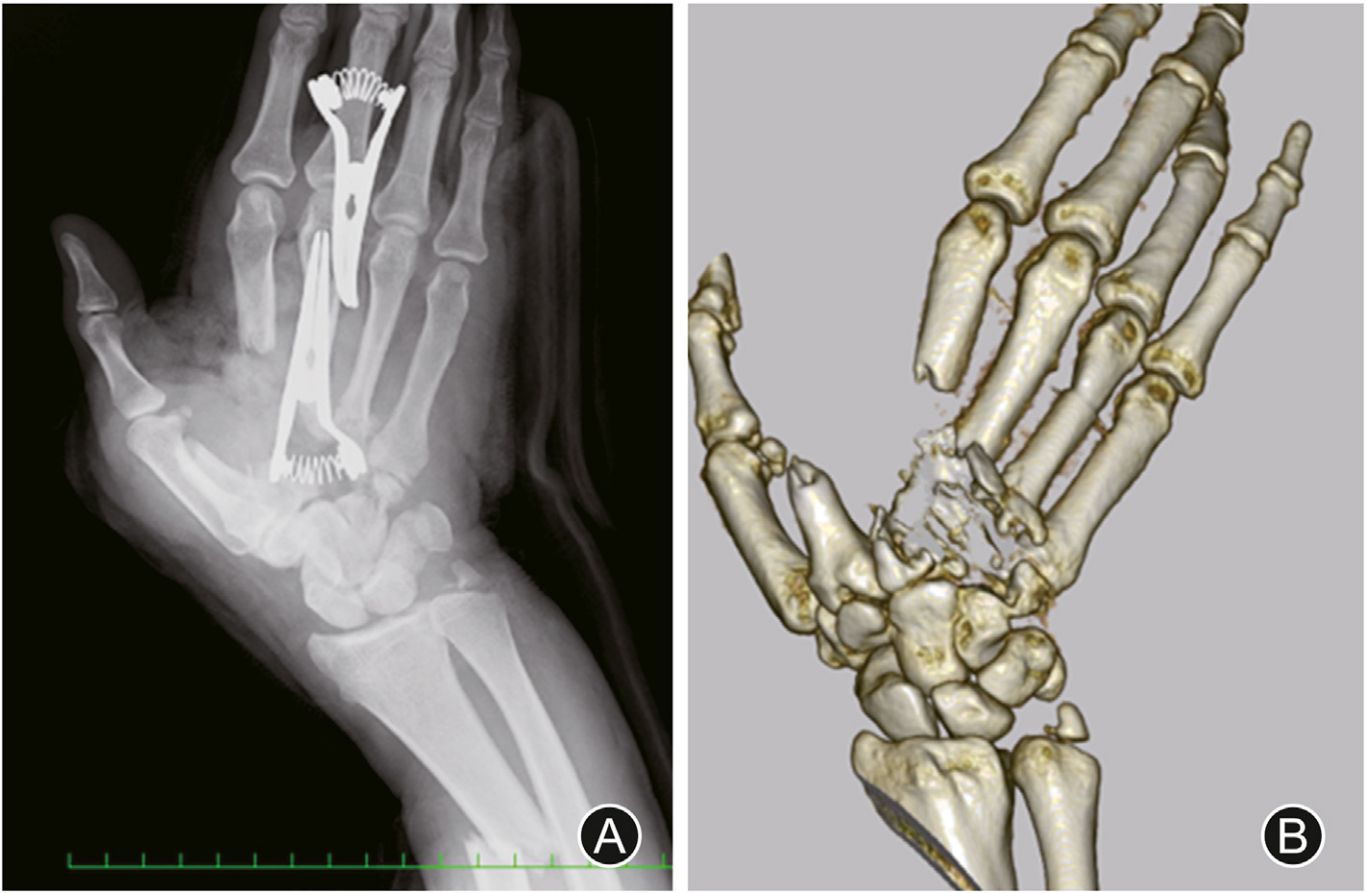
Images of the second, third, and fourth metacarpal bone fractures (A: radiograph, B: CT scan).
